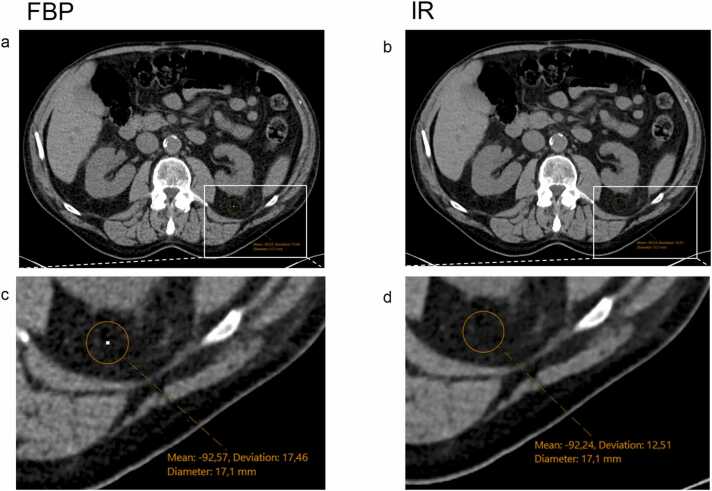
Exemplary CT slice (2D) of the abdomen at the L2/3 vertebral level without contrast medium, showing full abdominal views (a, b) and zoomed-in segments with density measurements (c, d). The images on the left were reconstructed using filtered back projection (FBP; a, c), the images on the right were reconstructed using iterative reconstruction (IR; b, d). Notably, the adipose tissue on the reconstructed with IR (right side, b and d) has a more homogeneous structure with lower density variations (lower noise). FBP=filtered back projection; IR=iterative reconstruction.
